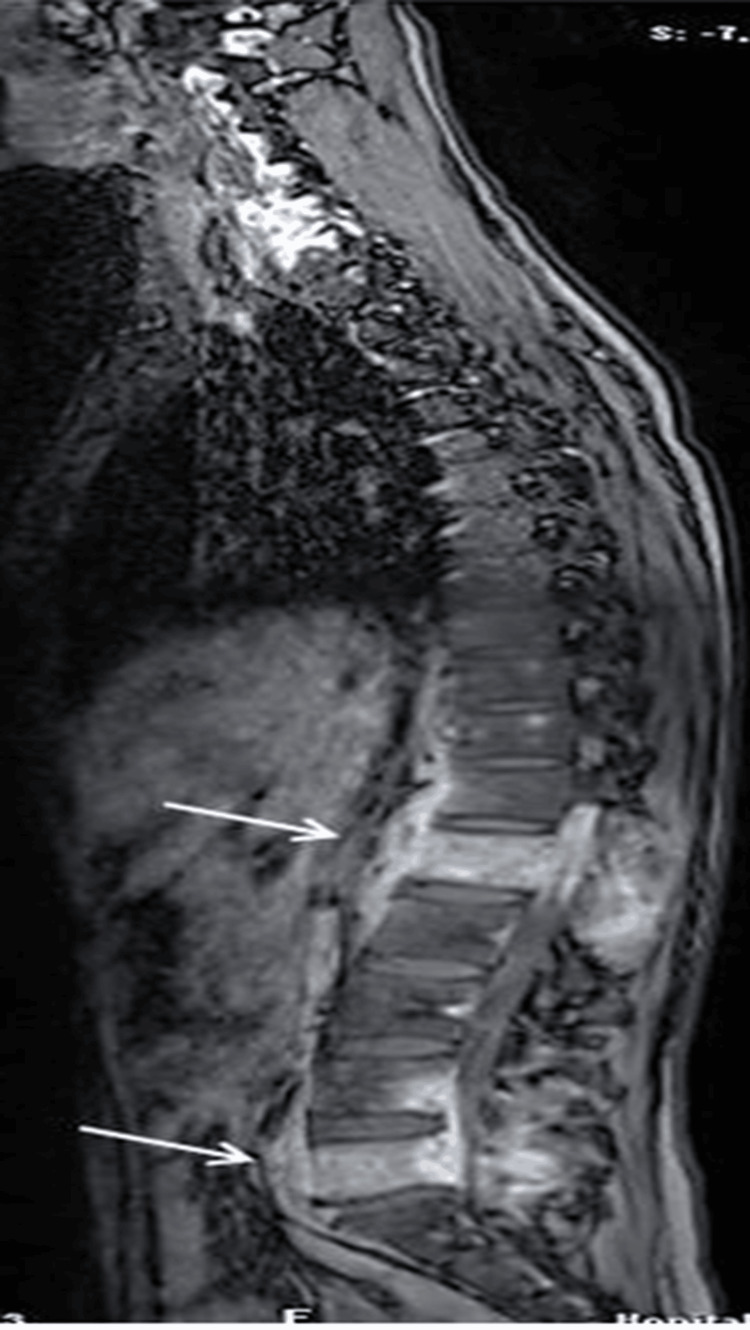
Sagittal view of magnetic resonance imaging showing inflammatory involvement of the vertebral body of the L1 and the posterior arches with large left paravertebral abscesses At L5 level: paravertebral abscesses with compression of the cauda equina nerve roots.
